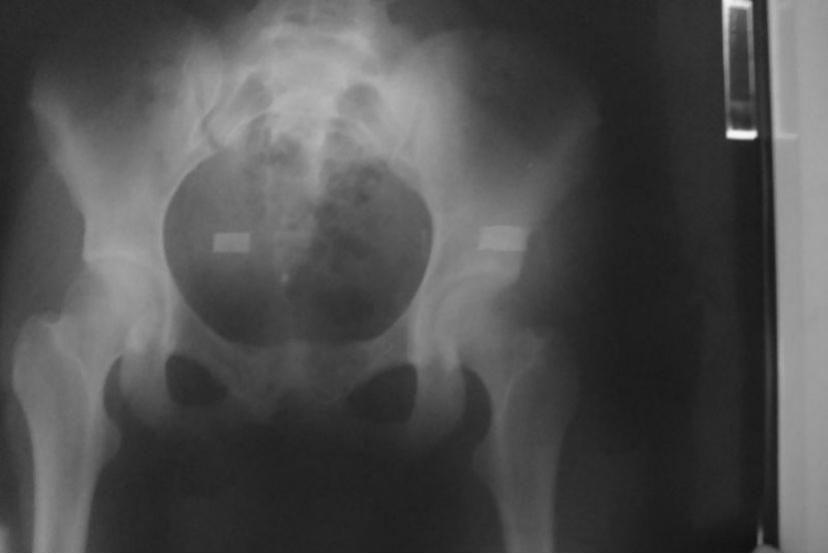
Post-operative X-ray following plate removal: maintained reduction in pubic symphysis with bone bridge and the closure of both triradiate cartilages
